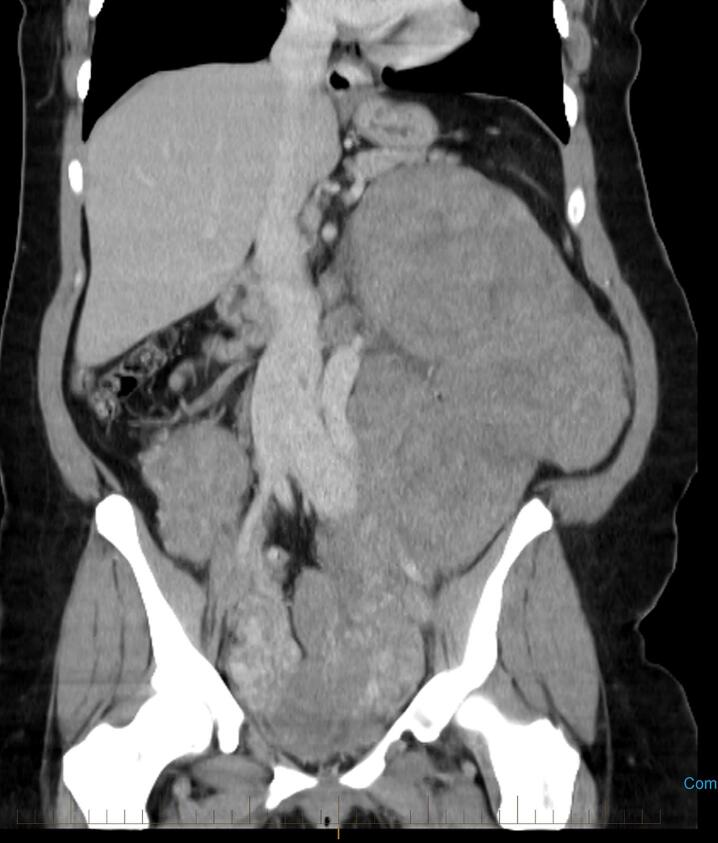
CT scan obtained at presentation, coronal view demonstrating a mass spanning from the pelvis to left upper quadrant.
